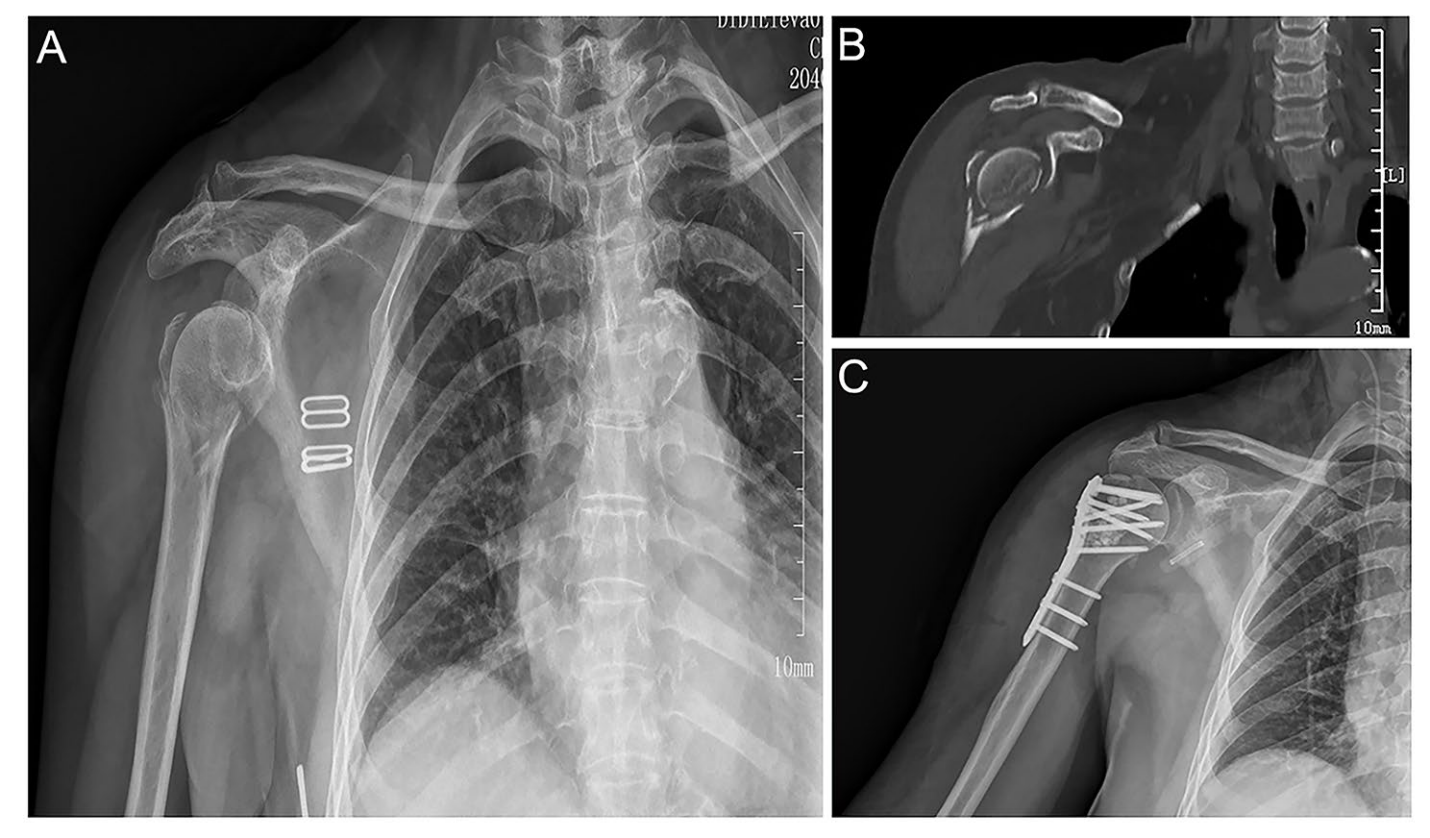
An example case of a right proximal humerus fracture in a 65-year-old woman treated with locking plate internal fixation. (A) X-ray images showing the fractures in the anatomical neck of the right humerus and the greater tuberosity, as well as valgus cottage and greater tuberosity displacement; (B) CT scan of the fracture and displacement; (C) Anterior posterior X-ray image showing good reduction of the anatomical neck and greater tuberosity fracture. CT, computed tomography
